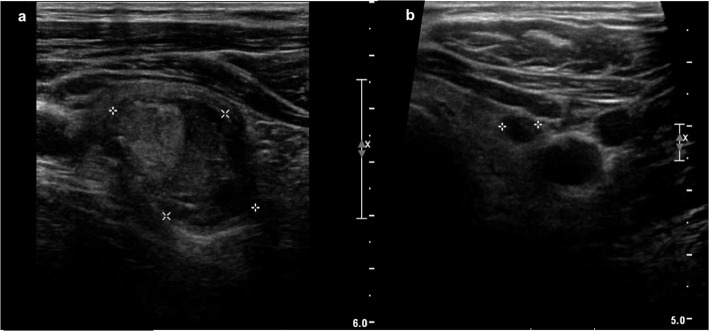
Thyroid ultrasound. a. Dominant 3.3 × 2.2 × 1.8 cm right thyroid nodule with heterogeneous echogenicity, mixed solid and cystic composition, lobulated borders, without echogenic foci, and without increased vascularity. b. One of several sub-centimeter left thyroid nodules that were hypoechoic, cystic, wider than tall, with smooth margins, without echogenic foci, and without increased vascularity
